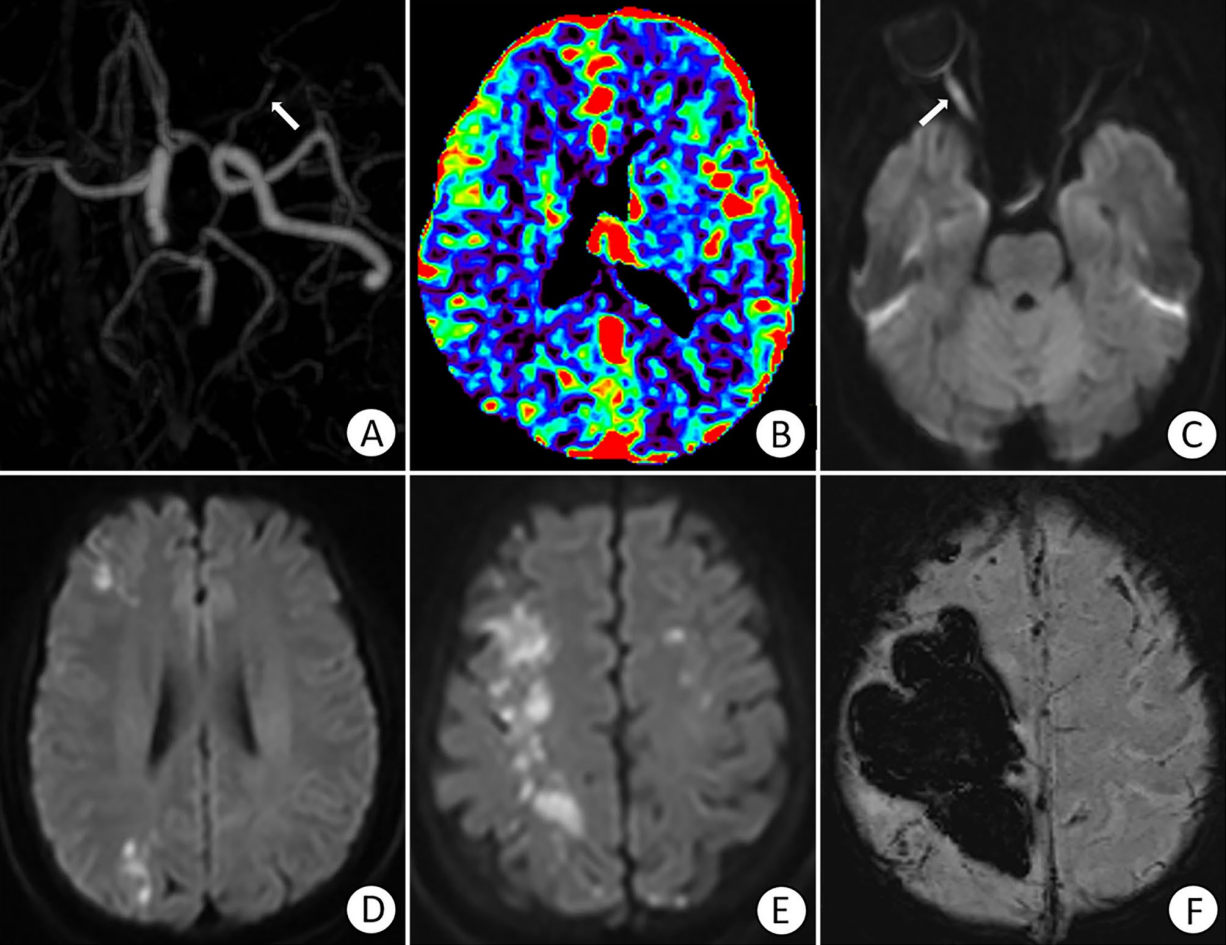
(A) CTA image of a patient 7.5 h after filler injection. The white arrow shows the normal left ophthalmic artery, while the right ophthalmic artery cannot be observed, reflecting its occlusion. (B) CT perfusion image showing that the time to peak perfusion was normal. (C) Diffusion-weighted imaging after 20 h showing a segmental hyperintense lesion in the thickened optic nerve of the right eye. (D, E) Diffusion-weighted imaging showing watershed infarctions in the bilateral frontal and parietal lobes and right occipital lobe. (F) Susceptibility-weighted image obtained after two days indicating postinfarction haemorrhage in the right cerebral hemisphere.
